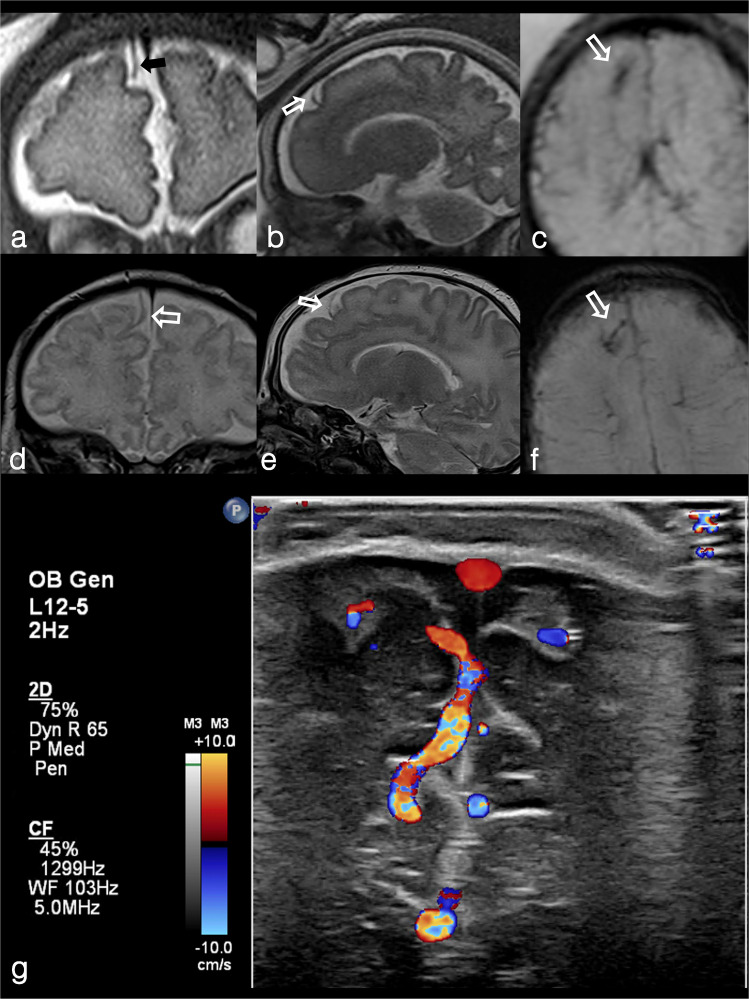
Magnetic resonance images of a developmental venous anomaly with superficial drainage. Magnetic resonance images in a male fetus (#6) at 33 weeks’ gestational age (a-c), postnatally at 24 days of life (d-f), and postnatal Doppler ultrasound at 54 days of life (g) demonstrate complicated developmental venous anomaly in the frontal lobe, with superficial drainage and parenchymal loss in the insertion of the draining vein. a, d Coronal and (b, e) parasagittal T2-weighted thin slices show the superficial vein transversing the right frontal parenchyma (*arrow*) with parenchymal loss in the insertion of the developmental venous anomaly. c, f Transverse susceptibility-weighted image shows blooming artifacts corresponding to the developmental venous anomaly (*empty arrow*). The classic “Caput Medusa” is shown only on the postnatal susceptibility-weighted imaging MRI (f). g Coronal postnatal Doppler ultrasound at 54 days of life demonstrates the insertion of the draining vein in the right frontal lobe
